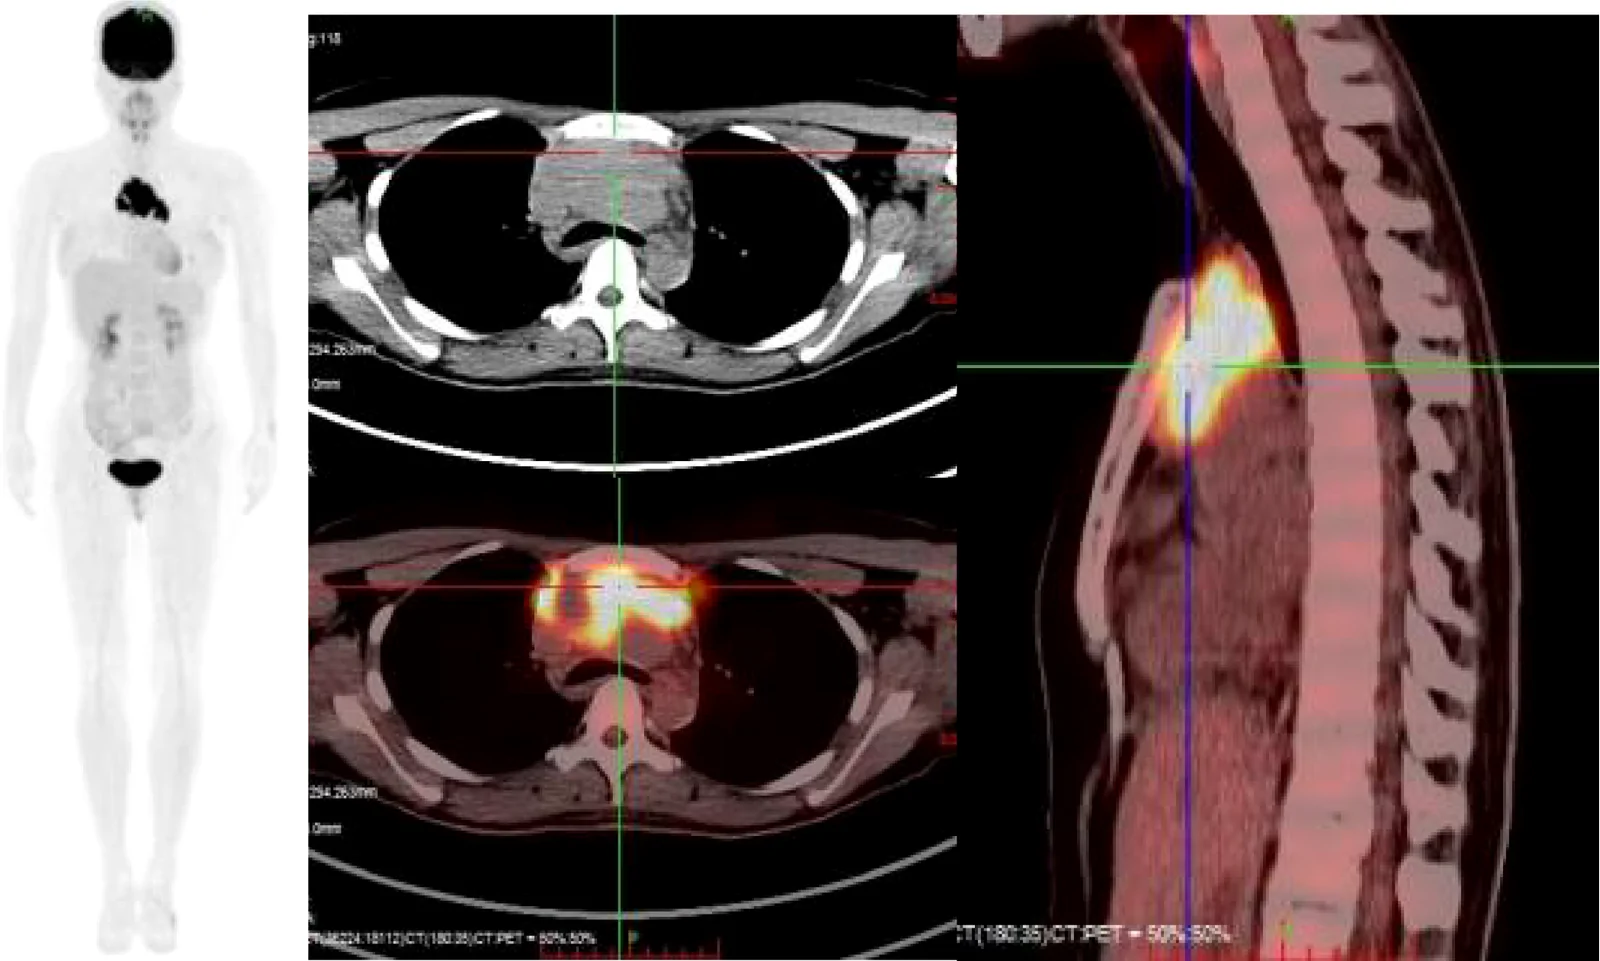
Whole-body MIP image demonstrates intense FDG uptake (SUVmax 20.0) localized to the anterior mediastinum, with physiologic background activity elsewhere. Axial PET-CT fusion image shows a 6.8 × 3.4 cm heterogeneous hypermetabolic mass encasing adjacent mediastinal vessels.
